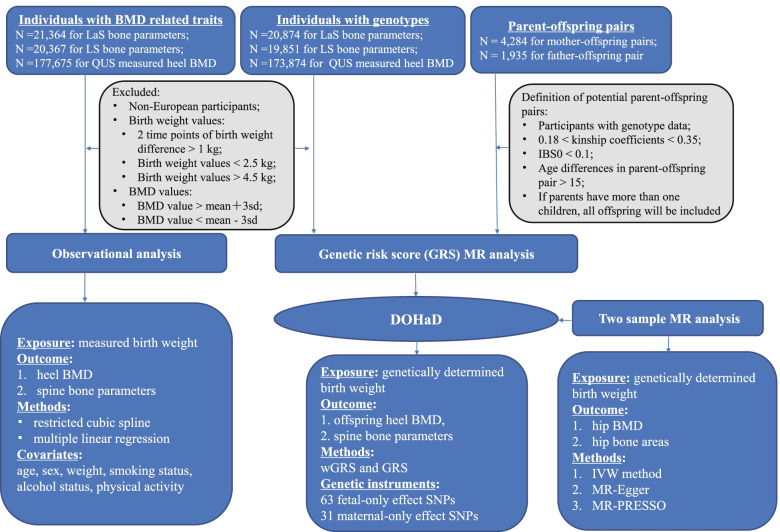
The study design overview. BMD, bone mineral density; IVW, inverse variance-weighted method; MR, Mendelian randomization; MR-PRESSO, MR pleiotropy residual sum and outlier test; QUS, quantitative ultrasound; DXA, dual-energy X-ray absorptiometry
